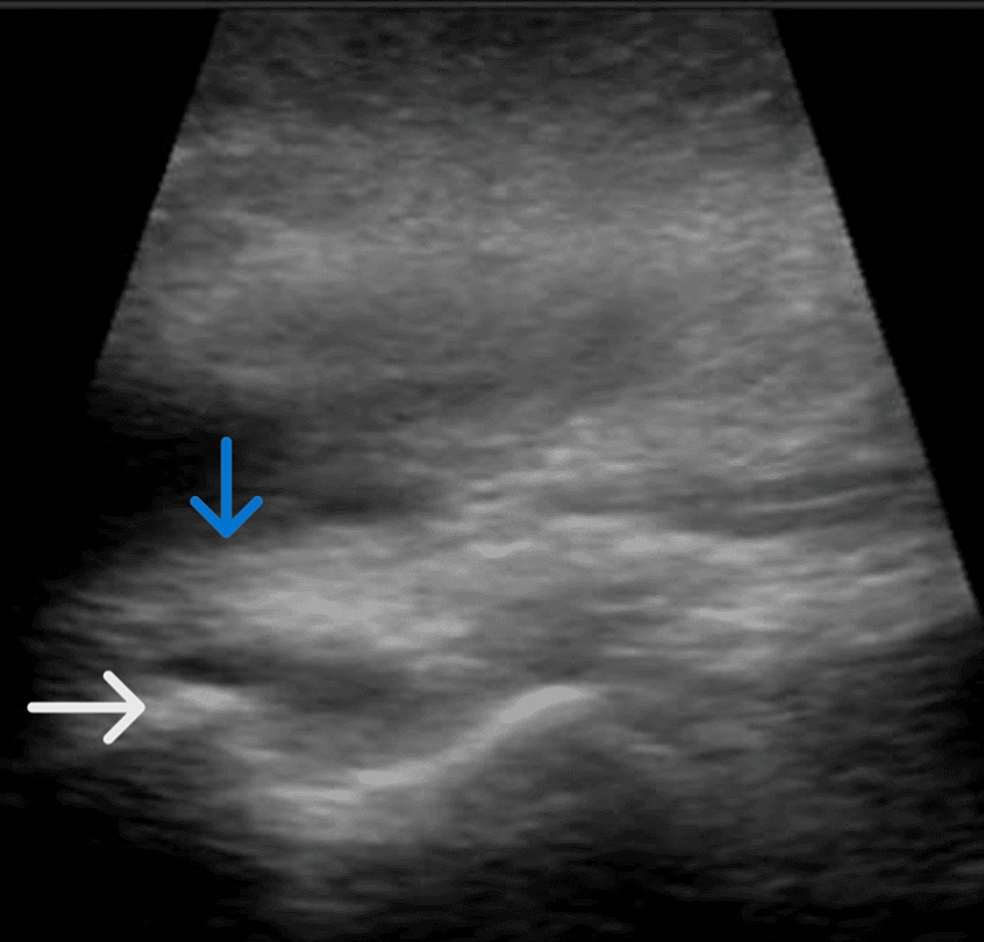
Bedside portable ultrasound demonstrating the humeral head (white arrow) in normal alignment with the scapular spine (blue arrow), indicating the successful reduction of the anterior dislocation
